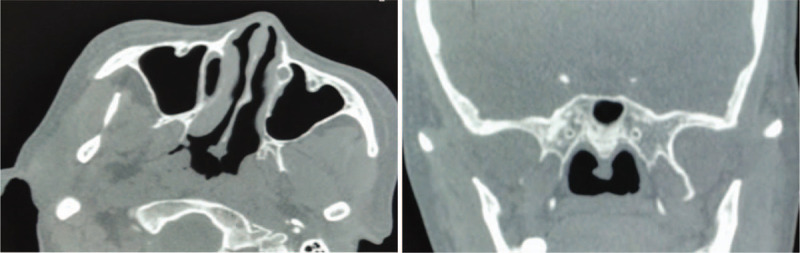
Computed tomography showing a soft tissue shadow on the caudal nasal septum.
